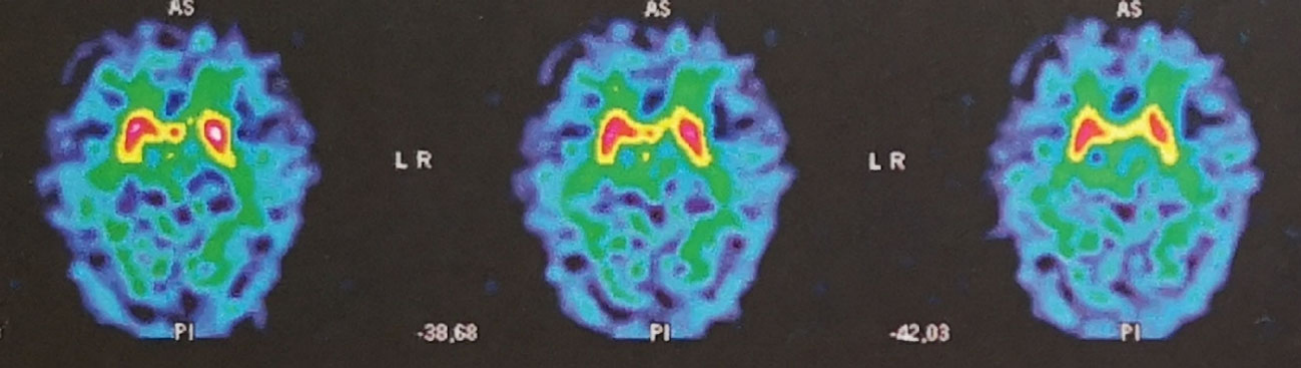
DaT-SCAN SPECT from patient #2, showing bilateral reduced striatal uptake, predominant in putamen, in favor of presynaptic dopaminergic denervation.
